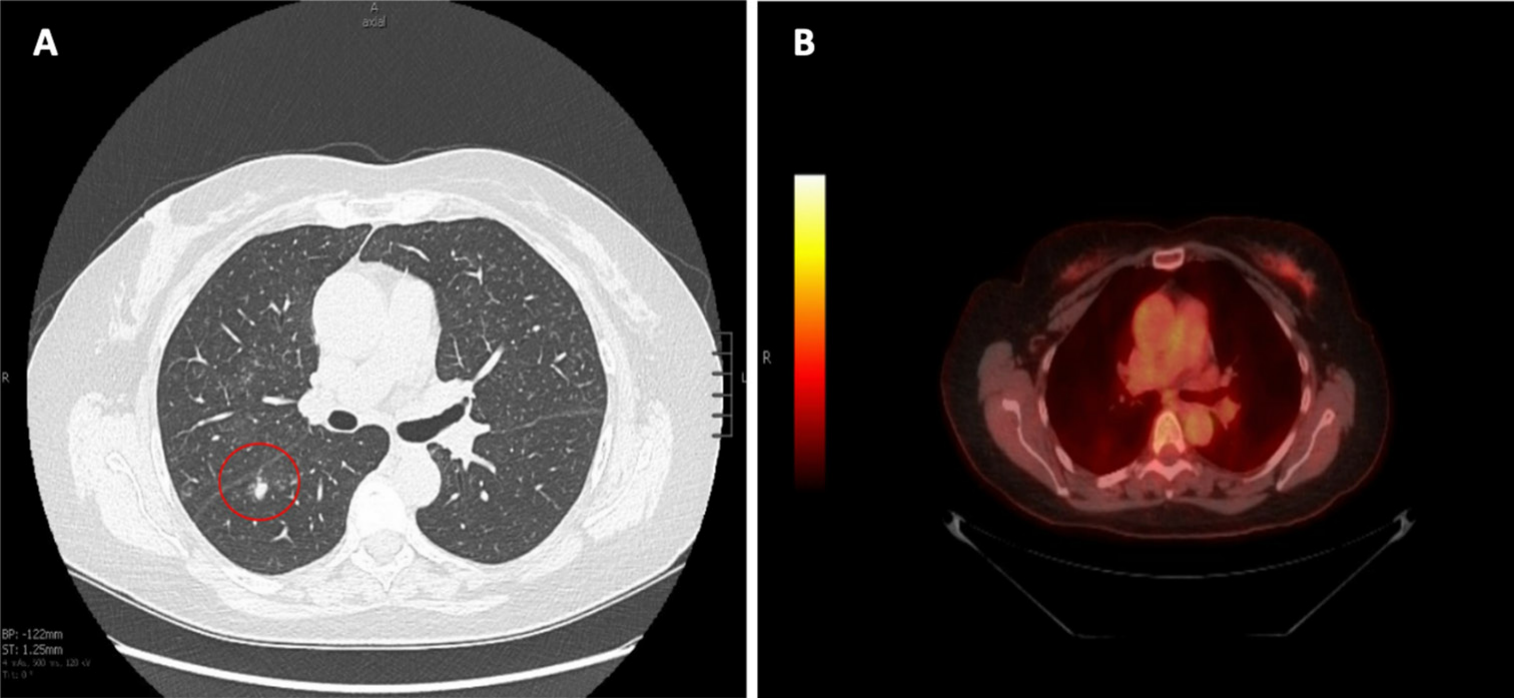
(A) Initial computed tomography (CT) scan of chest (axial view) showing faint upper lobe ground glass opacities and prominent right lower lobe (RLL) solid 6 mm nodule with surrounding ground glass; no lymphadenopathy seen. (B) Positron emission tomography (PET) scan with no fluorodeoxyglucose uptake at site of lesions.
